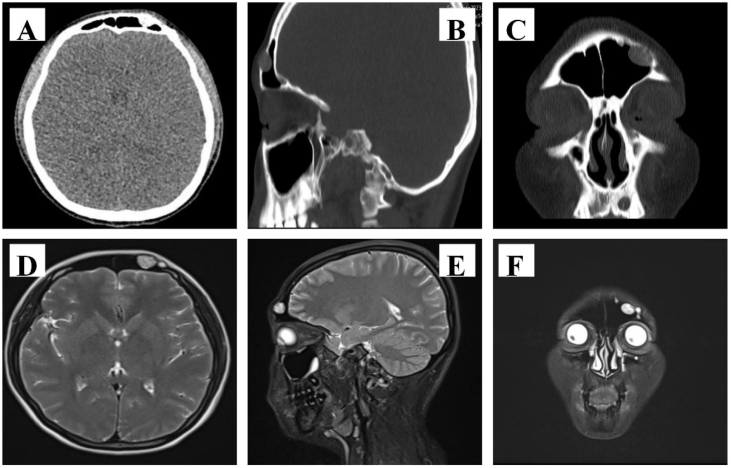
CT of the sinuses showed a frontal sinus mass (A, Position of cross section; B, Sagittal section; C, Coronal section). MRI of the sinuses showed a frontal sinus mass (D, Position of cross section; E, Sagittal section; F, Coronal section).
